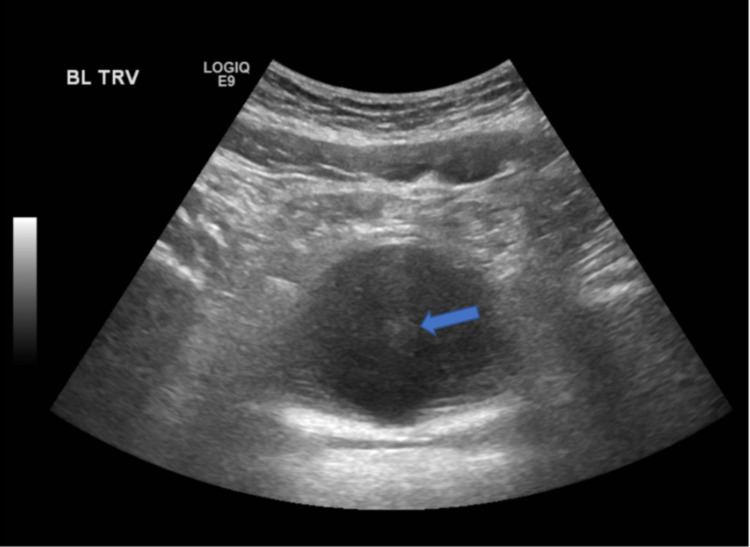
Post-op ultrasound of the pelvis: Ultrasound pelvis showed an under-distended urinary bladder with Foley catheter (blue arrow) in place and the previously seen large hematoma is no longer visualized.
